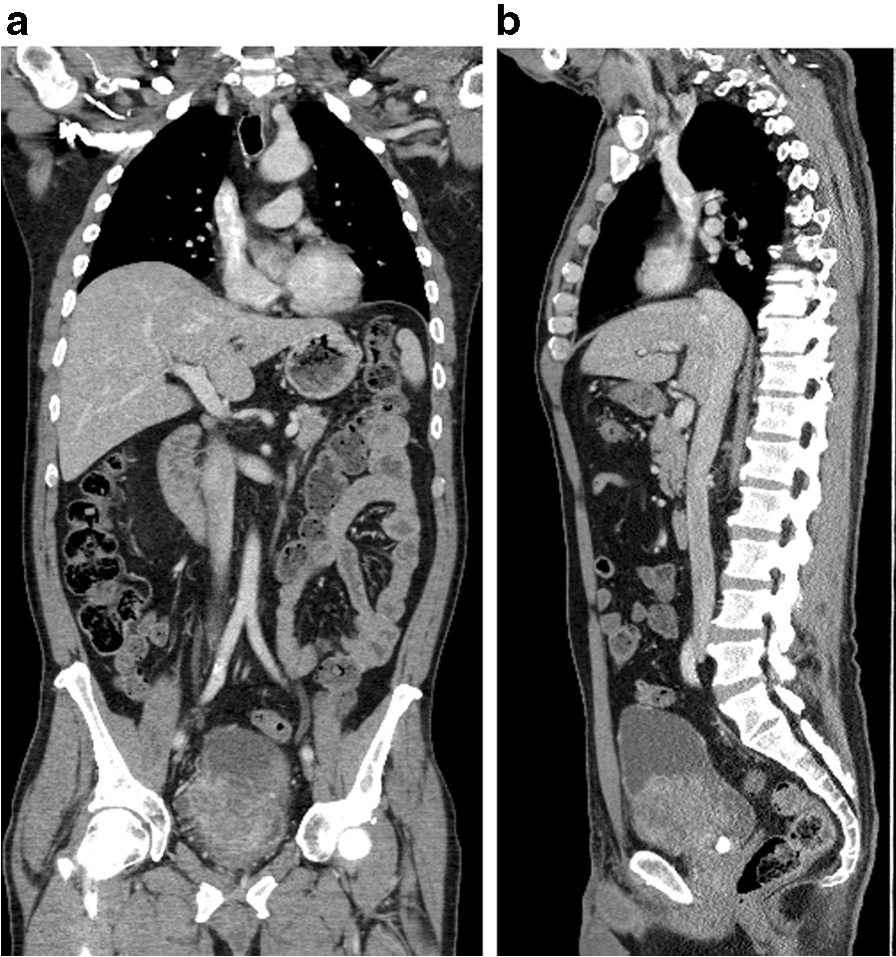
a Sagittal view of computed tomography scan of the thorax, abdomen, and pelvis showing the bladder tumor. b Coronal view of computed tomography scan of the thorax, abdomen, and pelvis showing the bladder tumor
